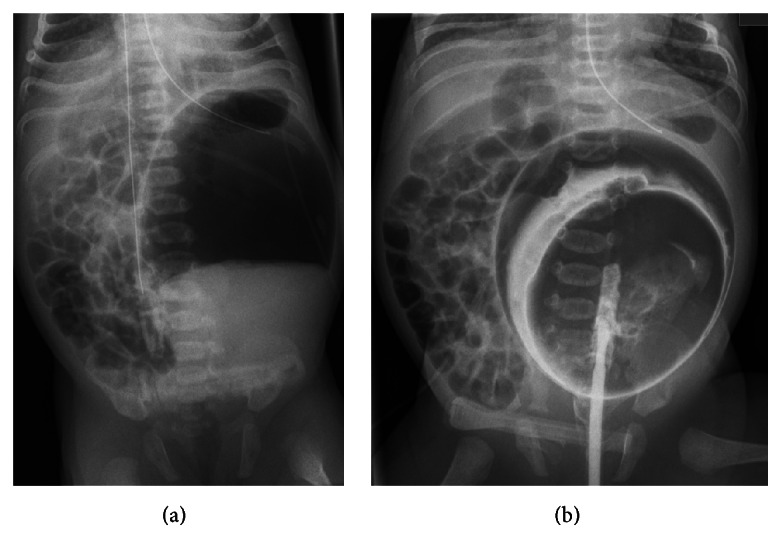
Upright anterior-posterior abdominal X-ray showing a big cystic lesion in the left hemiabdomen with complex contents (a); supine anterior-posterior abdominal X-ray after contrast enema (b).
